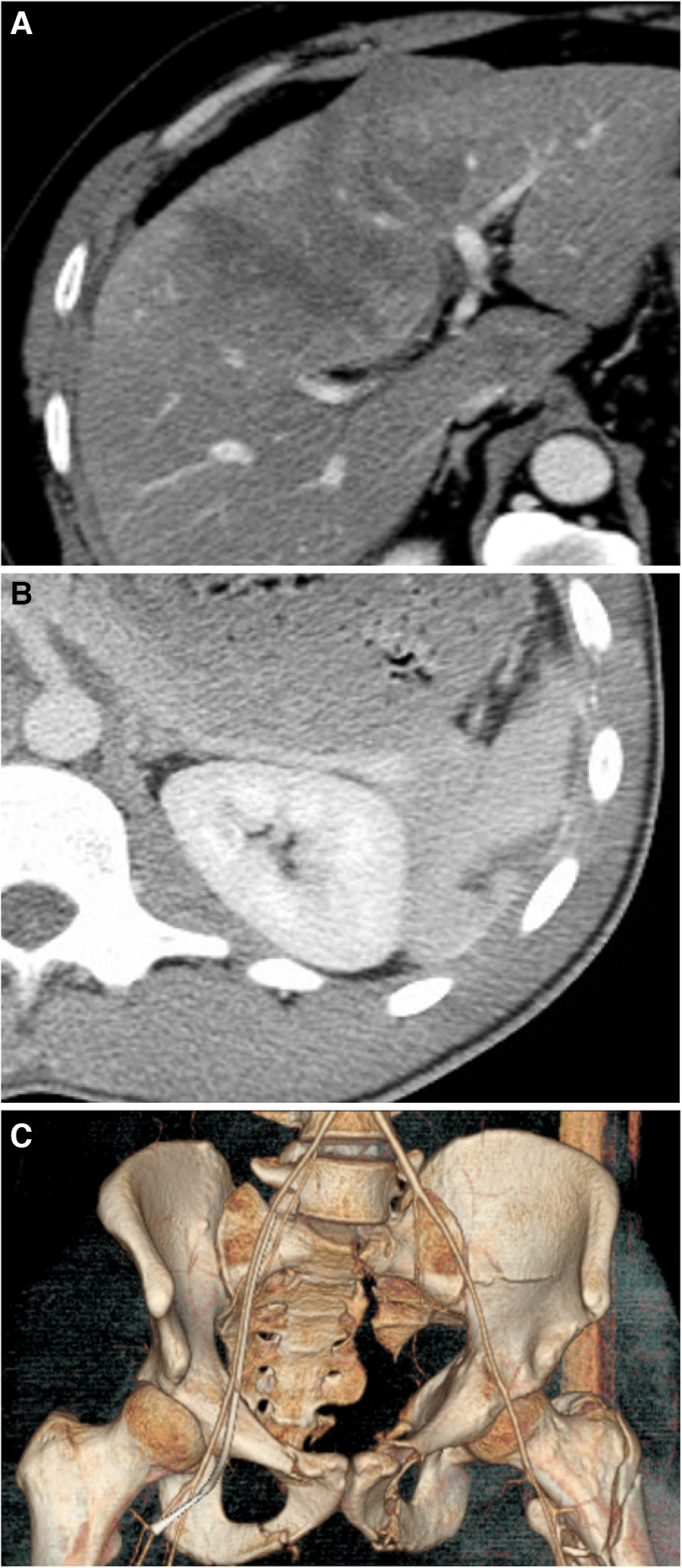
(A) A CT of the abdomen and pelvis showing a liver injury characterized by a subcapsular hematoma involving 10%–50% of surface area and an intraparenchymal hematoma measuring ≤10 cm in length (AAST grade II); (B) A CT of the abdomen and pelvis with contrast showing a splenic laceration of measuring less than 3 cm (AAST grade II); and (C) A 3D reconstruction of the pelvis of a 35-year-old man who fell from 5 stories showing multiple pelvic fractures, including the superior and inferior pubic ramus on the left, left acetabular fracture, and left moderately displaced sacral fracture, in addition to a left intertrochanteric fracture. There was also a suggestion of nondisplaced superior and inferior pubic rami fractures on the “right”.
